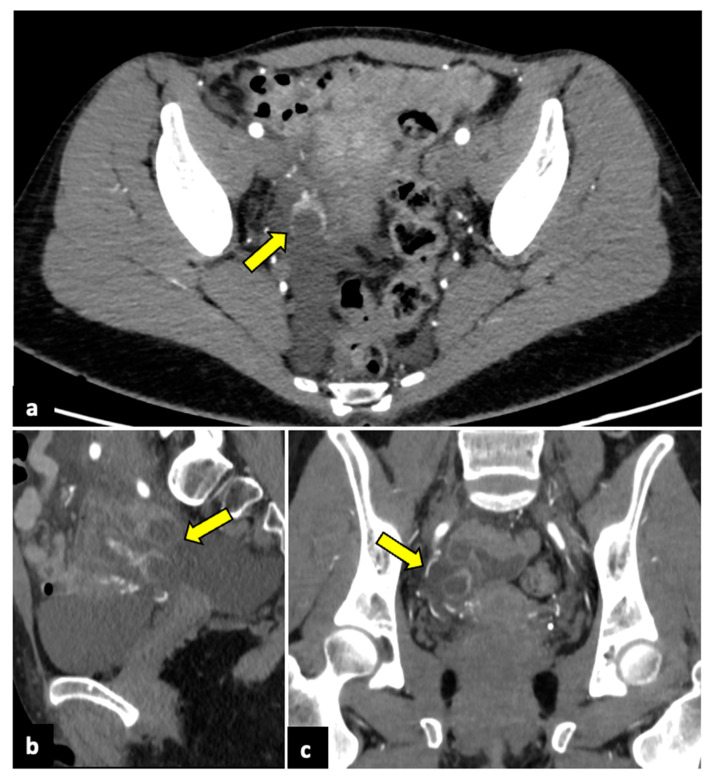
Ruptured ovarian corpus luteal cyst. CT axial (a), sagittal (b), and coronal (c) scans of a twenty-eight-year-old woman with acute right-sided pelvic pain. HCG test was negative. Images show a hypodense cystic lesion in the right adnexa, with thick and enhancing walls (yellow arrows). A focal discontinuity in the posterior wall is observed. Free pelvic fluid is also visible. The patient was treated conservatively.
